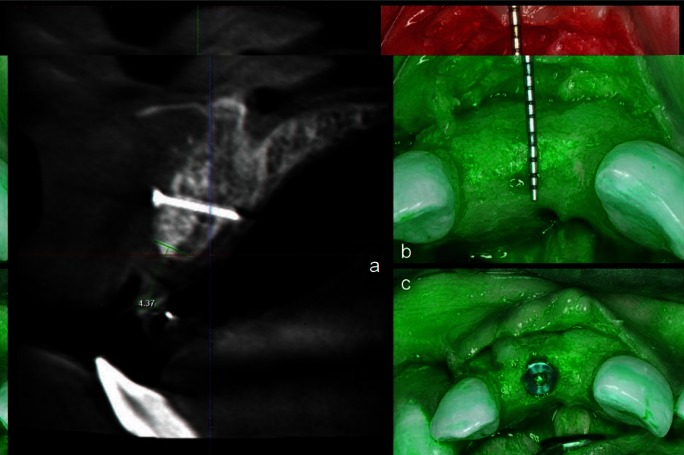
a) Six months post-operative radiographic (sagittal) view of augmented site. b) Clinical view of surgical site at re-entry after 6 months. c) Clinical view after implant placement.
